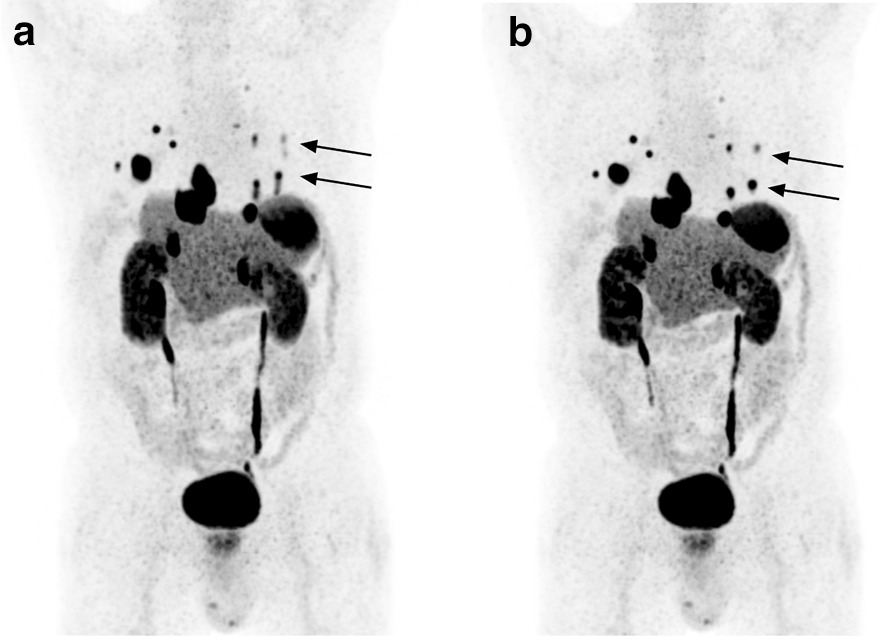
Anterior maximum intensity projections of a non-gated (a) and gated (b) [^68^Ga]Ga-DOTATOC PET examination in a patient with a rectal neuroendocrine tumour. Multiple lesions in the lung, with large blurring effects caused by respiratory motion are indicated by arrows. Both images have identical SUV_max_ grey scales of 0–15 g/mL
